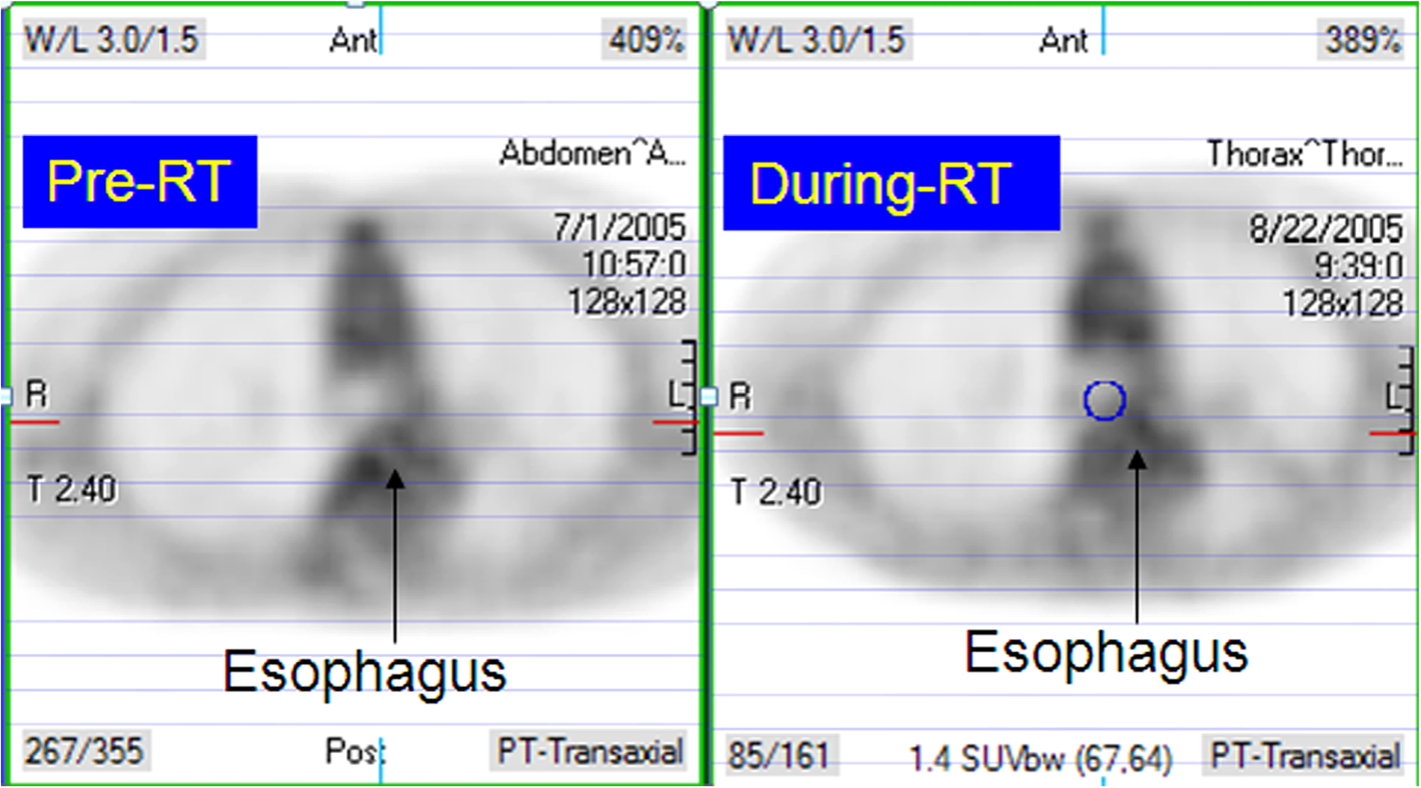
FDG-PET scans in patient without symptomatic esophagitis.
